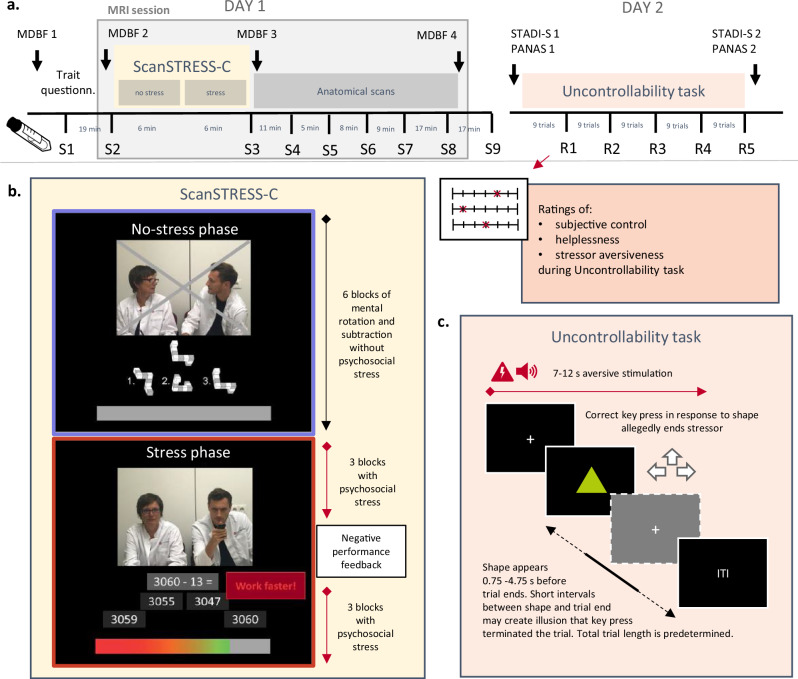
Experimental procedure.* a Overview of the experimental procedure with MRI session including the psychosocial stress task ScanSTRESS-C on day 1 and the uncontrollability task on day 2. b ScanSTRESS-C with demonstration of the two cognitive tasks that had to be solved (mental rotation and subtraction) in noStress and stress blocks. Jury not watching in the noStress phase but watching and criticizing slow and inaccurate performance in stress blocks, additional time limit indicated by the colored bar. c Uncontrollability task with aversive stimulation that is supposedly terminated by correct arrow key presses in response to a geometric shape. Trial termination is unrelated to key presses, but variable trial lengths can create the illusion of control over stimulation. S1-S9: Saliva samples; R1-R5: Ratings of subjective experience; MDBF: multidimensional mood questionnaire; STADI-S: State-trait anxiety and depression inventory state version; PANAS: positive and negative affect scale, ITI: Inter trial interval. b Reprinted and adapted from *Psychoneuroendocrinology*, 118, Sandner, M., Lois, G., Streit, F., Zeier, P., Kirsch, P., Wüst, S., & Wessa, M., Investigating individual stress reactivity: High hair cortisol predicts lower acute stress responses. 104660, Copyright Elsevier Ltd. (2020), with permission from Elsevier.
